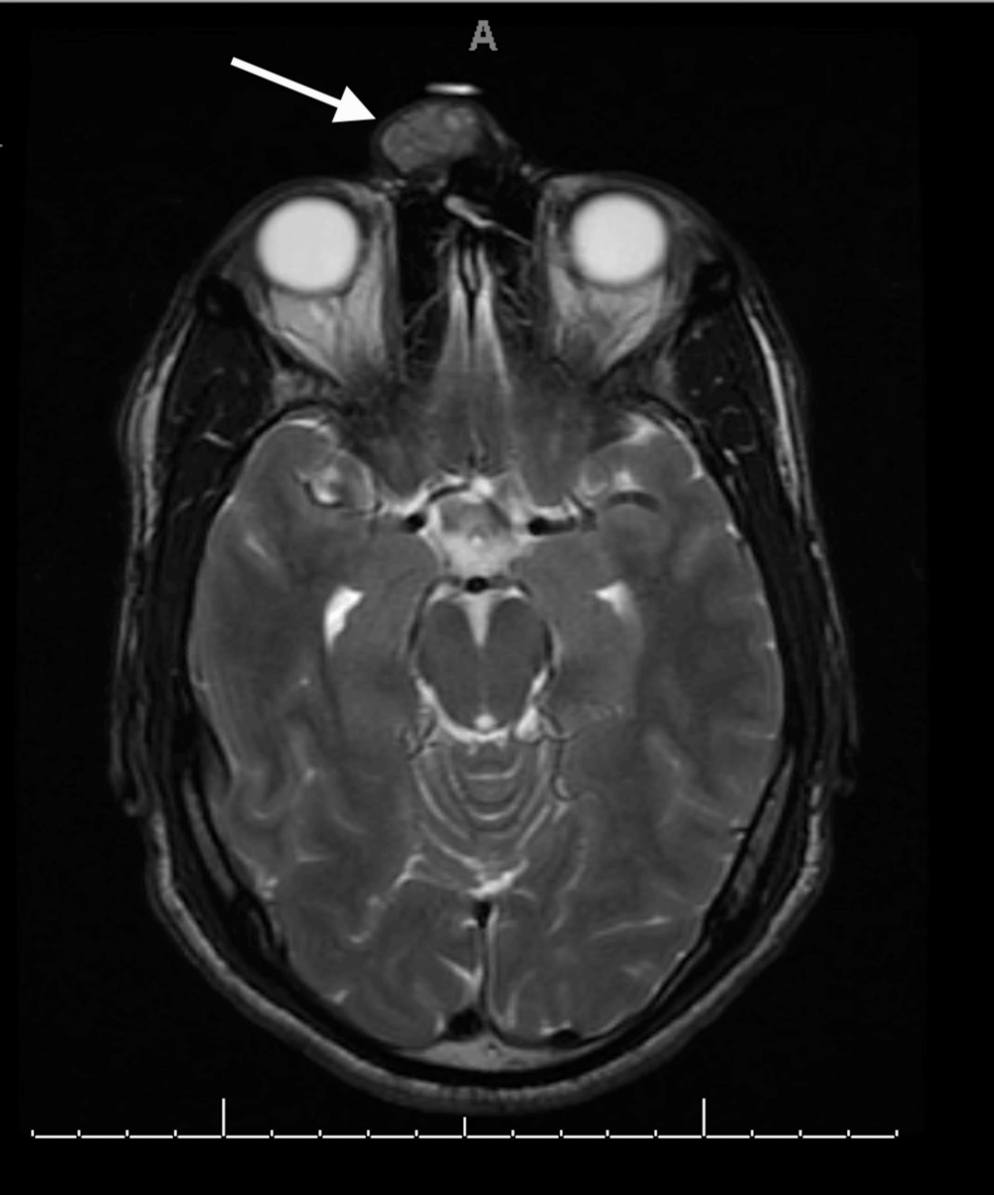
Preoperative MRI (pre-contrast T2-weighted) Preoperative MRI: axial cut of a pre-contrast T2-weighted image with an arrow demonstrating an isointense mass within the glabellar region
